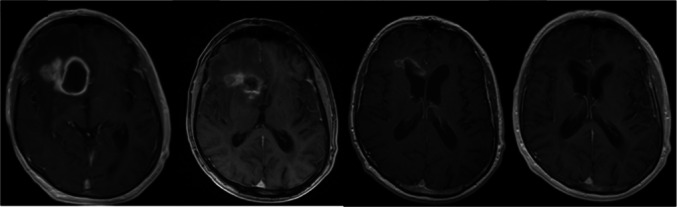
Sequential axial post-contrast T1- weighted MRI images obtained in a 49-year-old patient with left temporal brain abscess. A: Detection of the abscess formation, B: MRI performed on postoperative-day 1 after craniotomy, abscess evacuation and partial resection, C: MRI performed 28 days after initiation of HBOT showing further remission, D: MRI at 3-month follow up demonstrating no residual abscess
